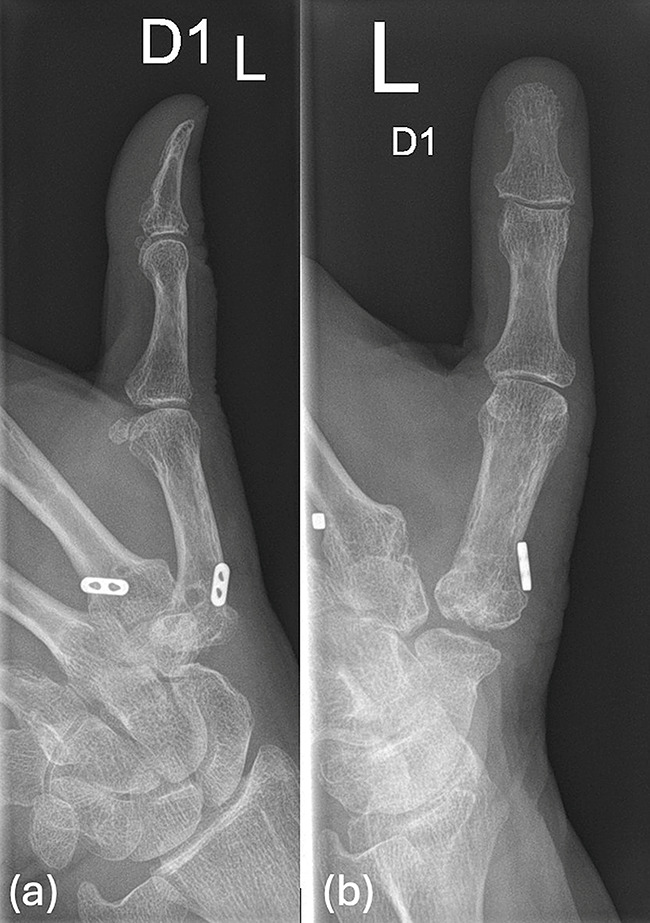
Radiographic views (a: lateral, b: frontal). Status after trapeziectomy and Tight Rope (Arthrex^®^) cable suspension. Significant subsidence of the thumb metacarpal in the resection space is evident. There is pronounced exostosis formation at the base of the first metacarpal bone. Distal scaphoid width was measured to be 9 mm
